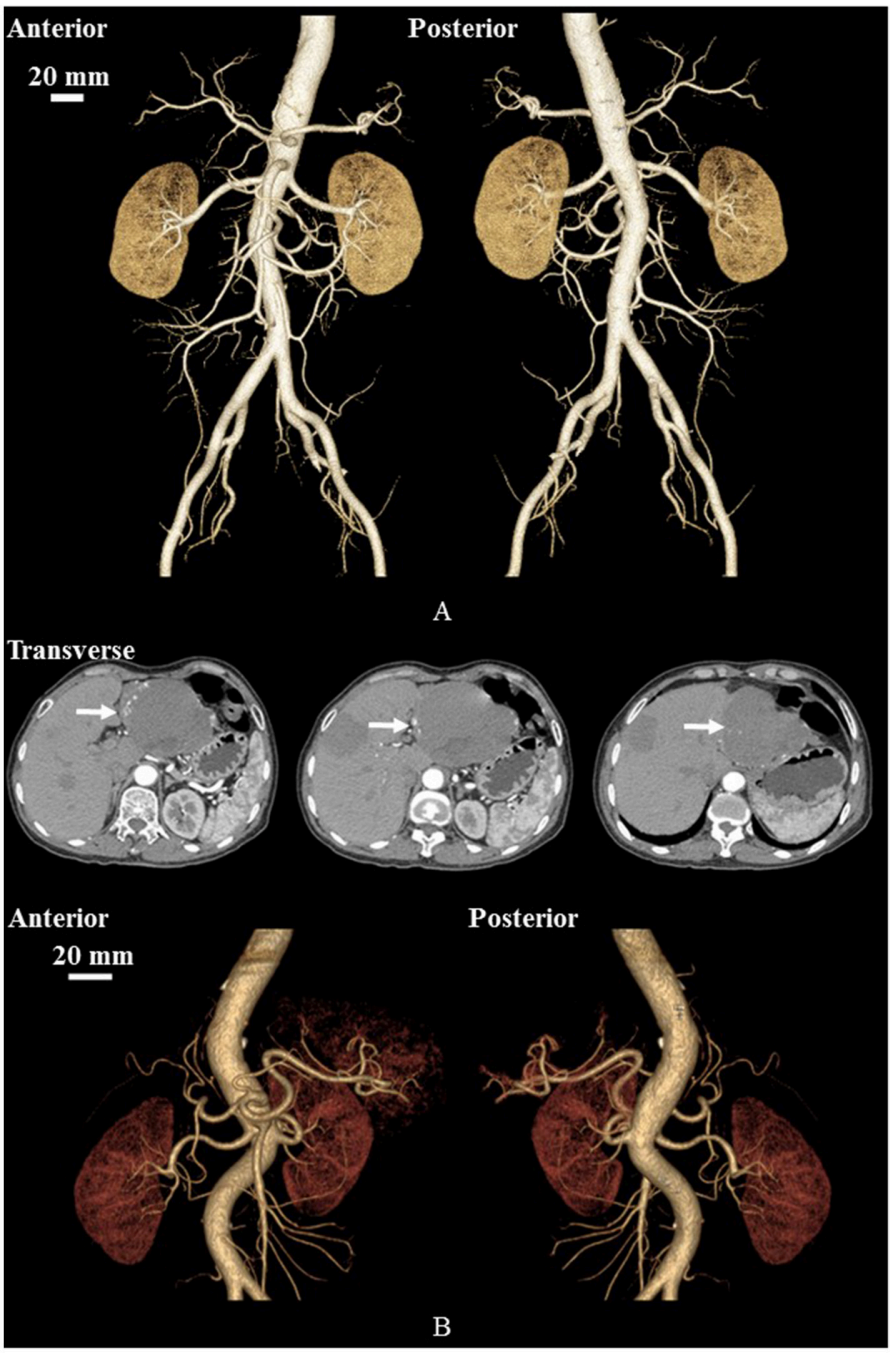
Geometrical models reconstructed from CT images of a representative control (A) and a representative HH patient (B). (B) Also shows CT-axial angiographs, where arrows mark liver hemangiomas.
